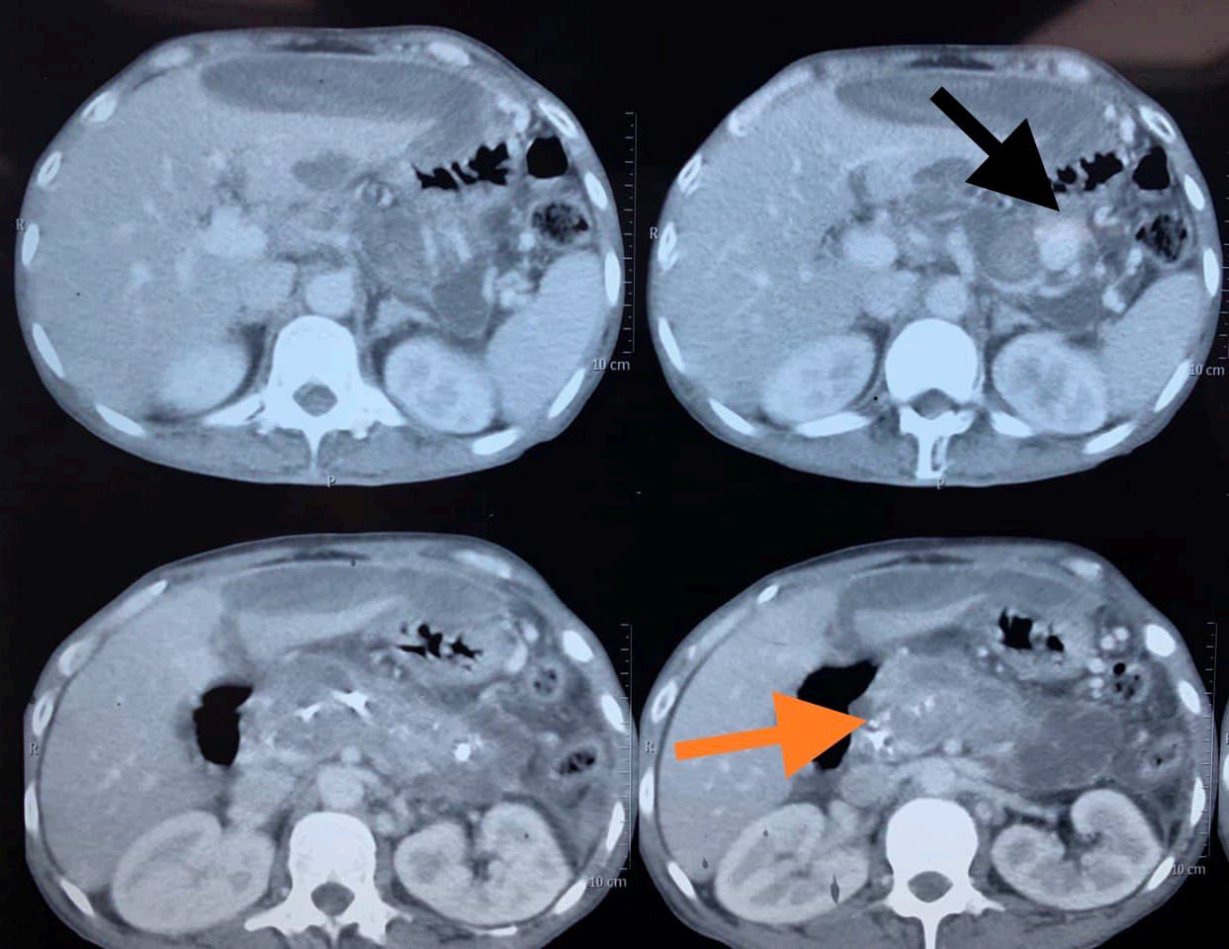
Axial sections from the contrast-enhanced computed tomography (CECT). Black arrow: splenic artery pseudoaneurysm arising from the distal third of the artery. Orange arrow: calcifications within the pancreatic parenchyma.
